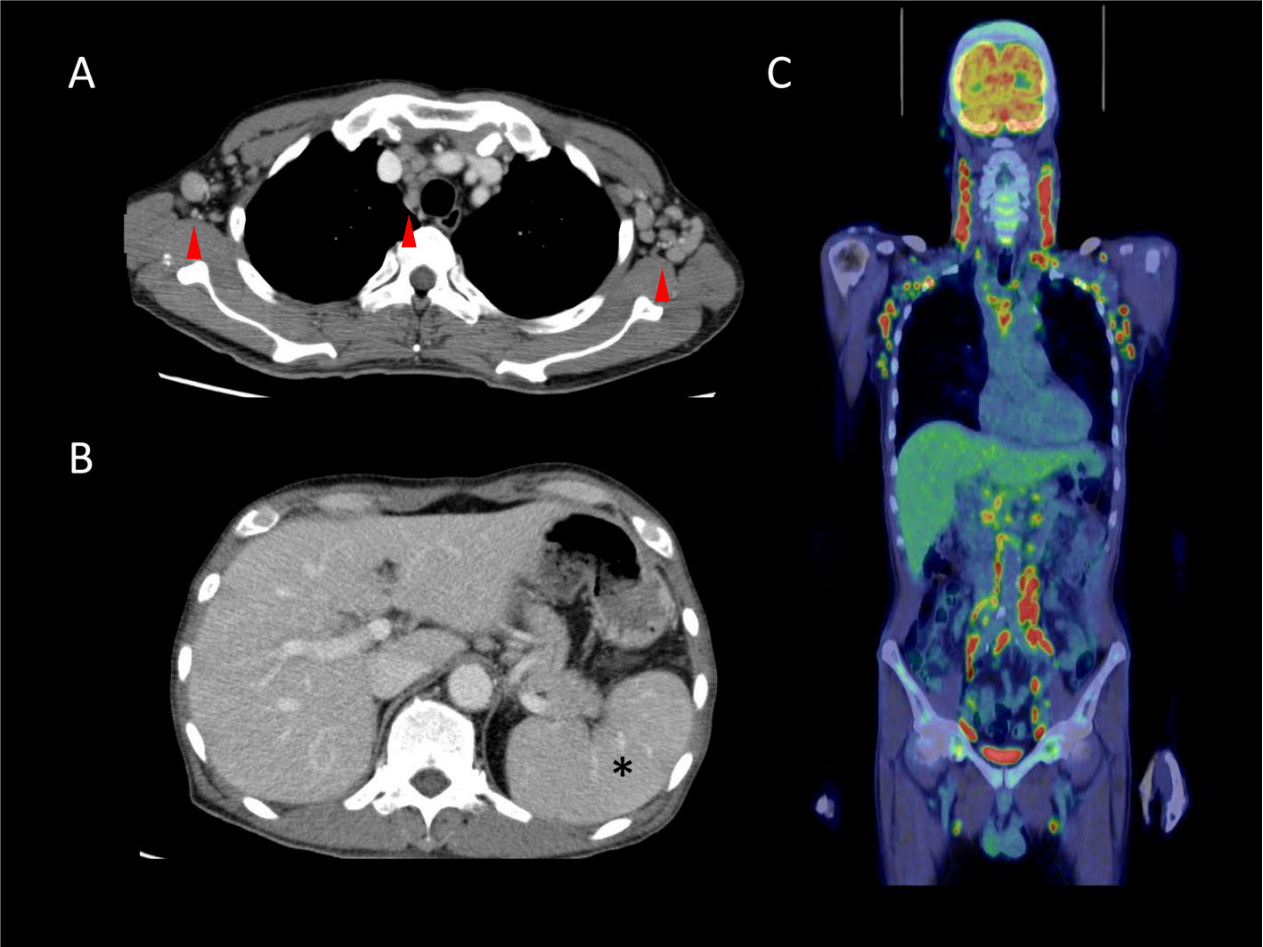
During the 2nd cycle of maintenance therapy following induction therapy with temozolomide, the patient experienced constitutional symptoms and cytopenia. Computed tomography showed systemic lymphadenopathy (A; arrowhead) and splenomegaly (B; asterisk). Positron emission tomography showed marked fluorodeoxyglucose-avid lymph nodes in the neck, axillae, mediastinum and para-aortic region (C)
